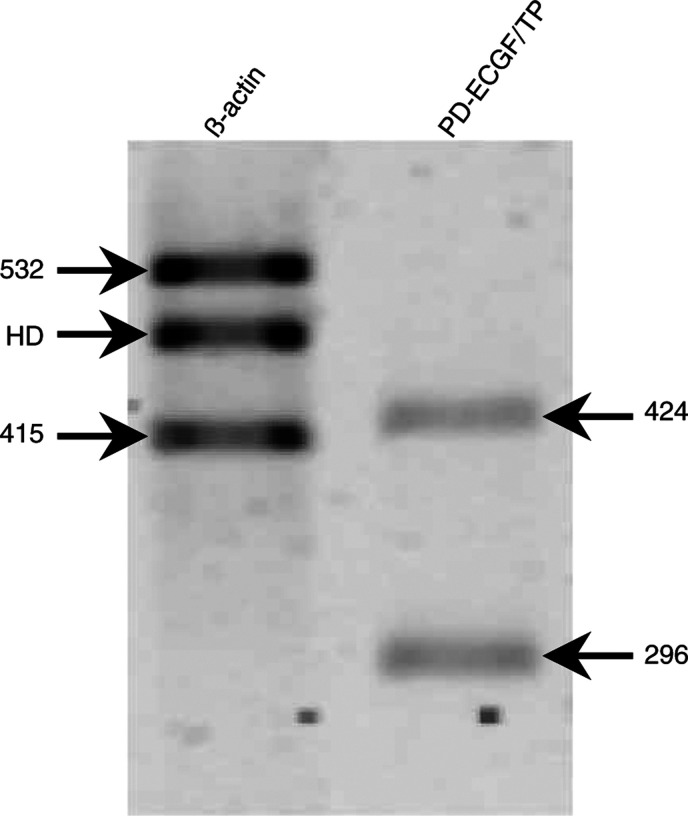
Representative example of an agarose gel on which PCR products are separated according to their expected sizes. The gels show three bands for *β*-actin: bands of 532 and 415 bp are encoded by the forward and reverse primer for the native cDNA and CT, respectively, the third band is the heteroduplex consisting of native cDNA and CT. For TP, only two bands are visible, the native cDNA of 424 and 294 bp for the CT, the heteroduplex was formed occasionally. The bands were scanned and the OD was used to calculate a ratio between the native cDNA and CT. The contribution of the heteroduplex was calculated as described previously (Willey *et al*, 1998; Rots *et al*, 2000).
